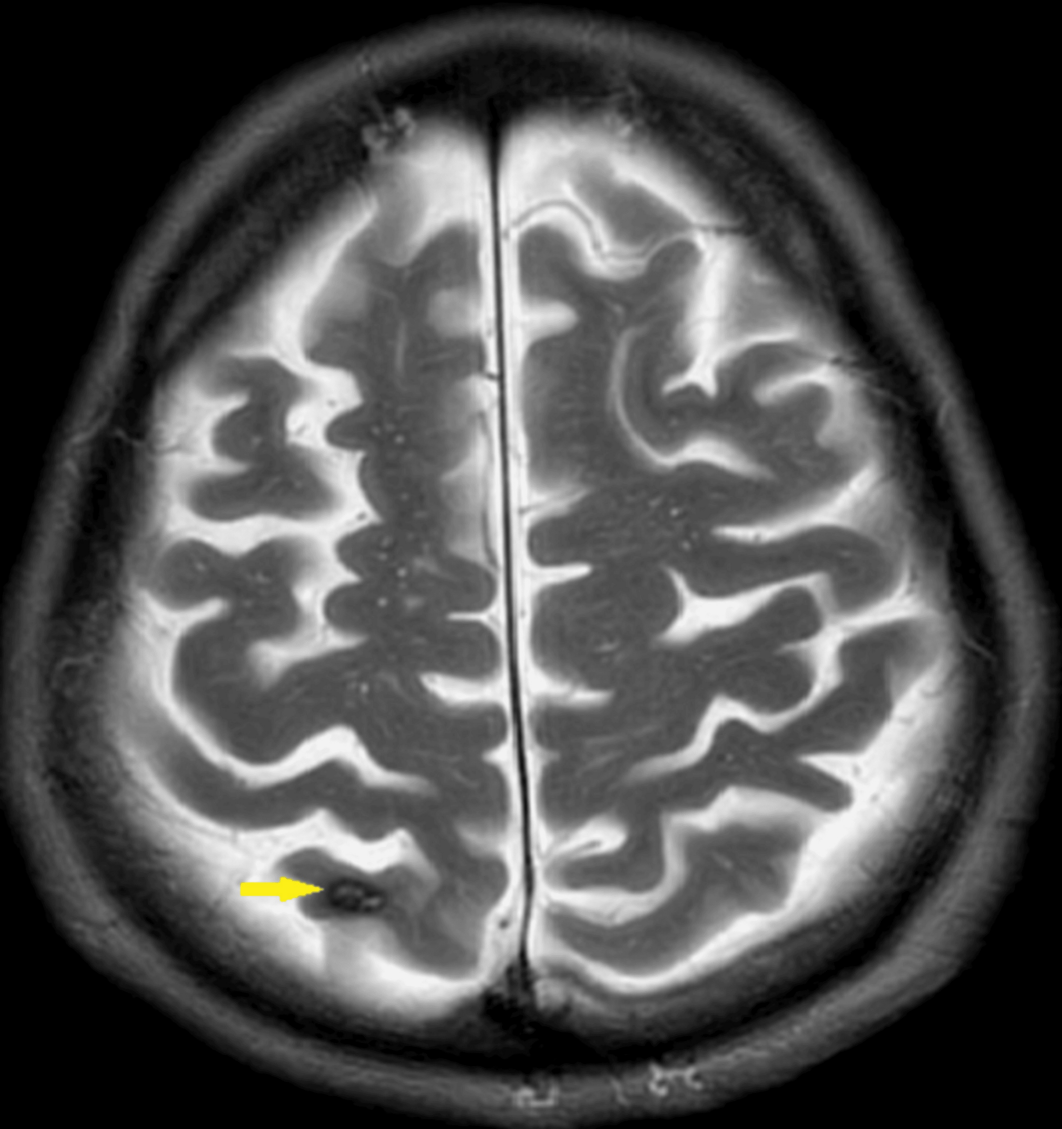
MRI head showing feature suggestive of cavernous hemangioma (yellow arrow).
